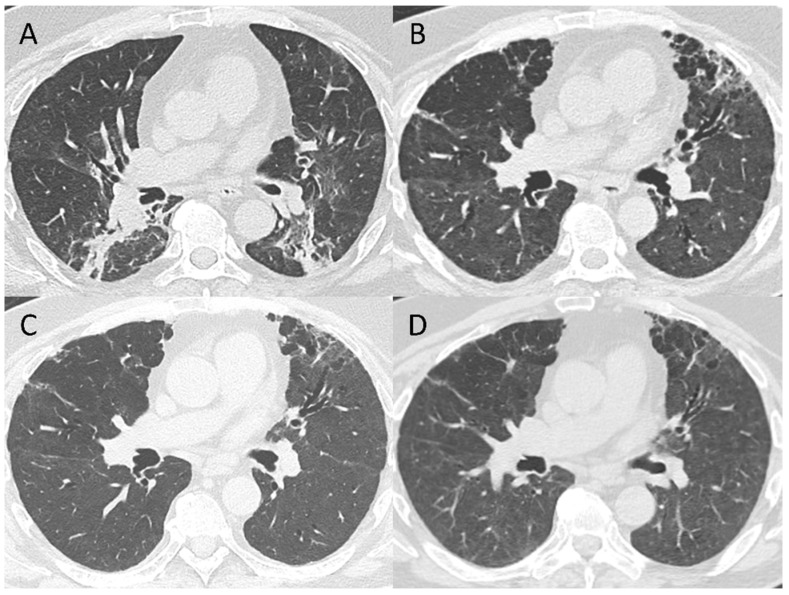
Representative CT images of post-ventilatory residual fibrotic abnormalities in a patient who received invasive mechanical ventilation. From baseline (A) to 3-month follow-up CT scan (B) a progressive resolution of GGO and consolidations at lower lobes can be observed, together with the appearance of bronchiectasis, GGO, and cystic spaces in the subpleural interface of the anterior part of the upper left lobe in keeping with post-ventilatory damage. These abnormalities are persistent at 6-month (C) and 12-month follow-up CT scans, even if a decrease in residual disease extension can be observed (D).
